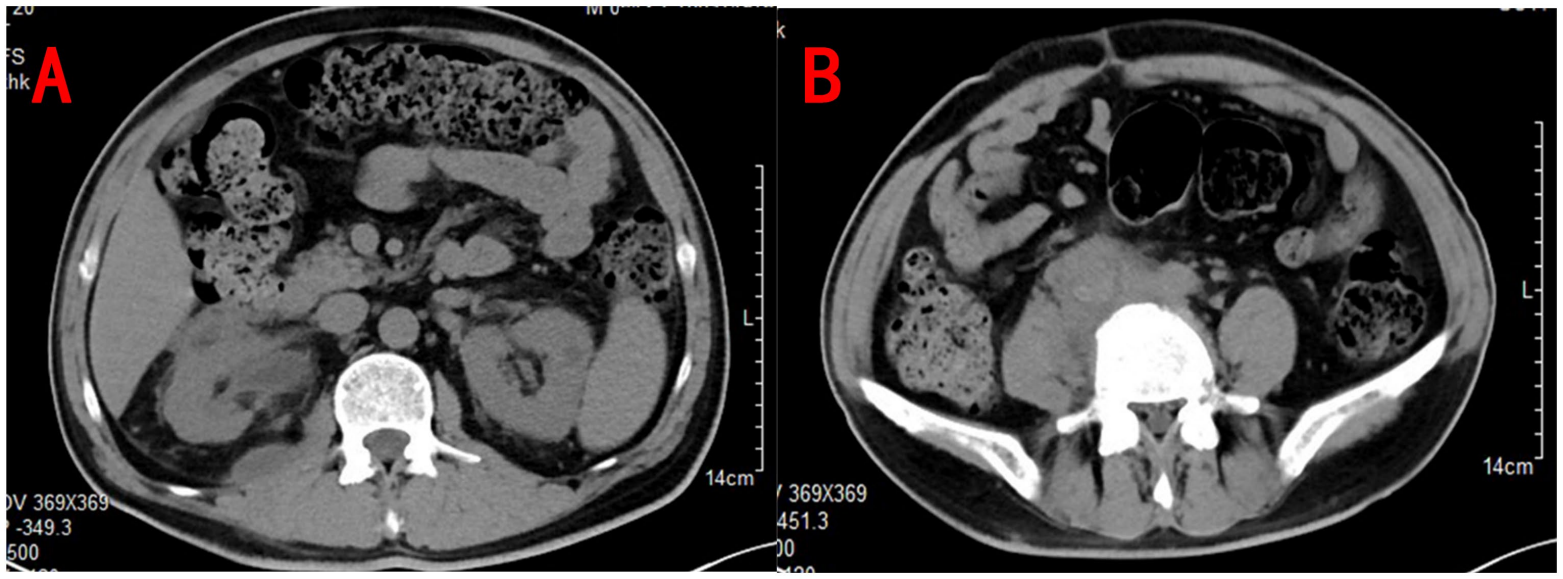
Urinary CT showed slightly more hydronephrosis in the right kidney and more perirenal inflammation than before (A), and soft tissue density shadows are seen around the inferior vena cava and the right common iliac vessels (B).
